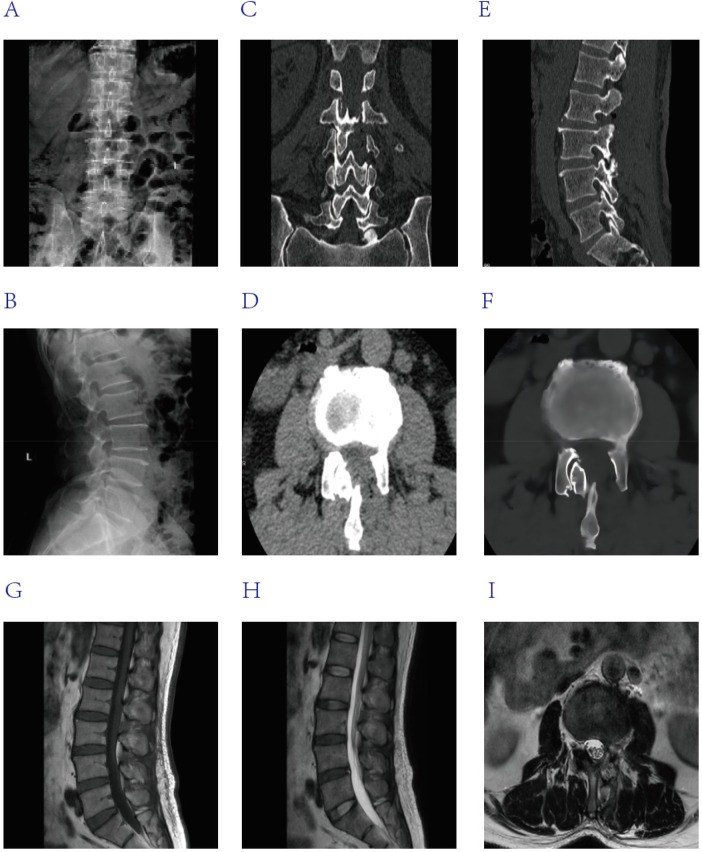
Postoperative follow-up imaging evaluation. (A,B) Postoperative lumbar spine hyperextension anteroposterior and lateral radiographs, demonstrating good spinal alignment and stability, with no evidence of abnormal motion. (C–F) Postoperative lumbar CT scans, including coronal, sagittal, and axial reconstructions, showing only limited partial laminectomy with well-preserved osseous structures, indicating minimal surgical trauma. (G–I) Postoperative lumbar MRI scans, including sagittal and axial sequences, confirming complete resection of the lesion, adequate spinal canal decompression, and no evidence of residual or recurrent disease.
